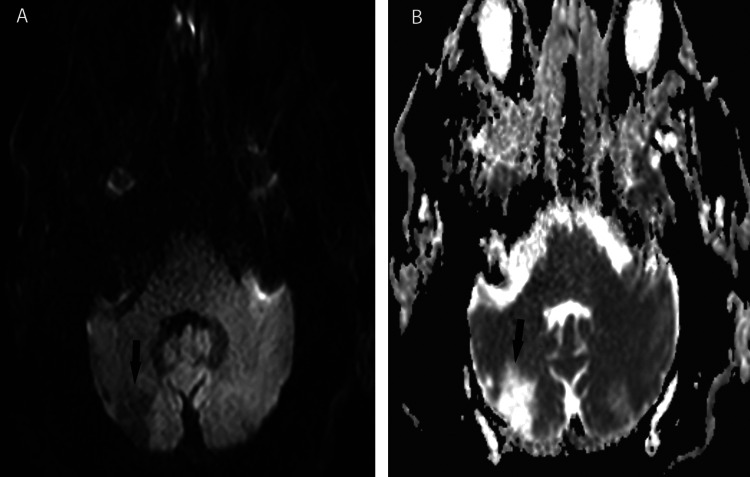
Follow up MRI A. Trace image. B. ADC image. Arrows show chronic right cerebellar hemisphere stroke, with no evidence of acute ischemia.  Hyperdense areas on the ADC image in the left cerebellar hemisphere were considered artifact as they did not appear on other MRI sequences.
